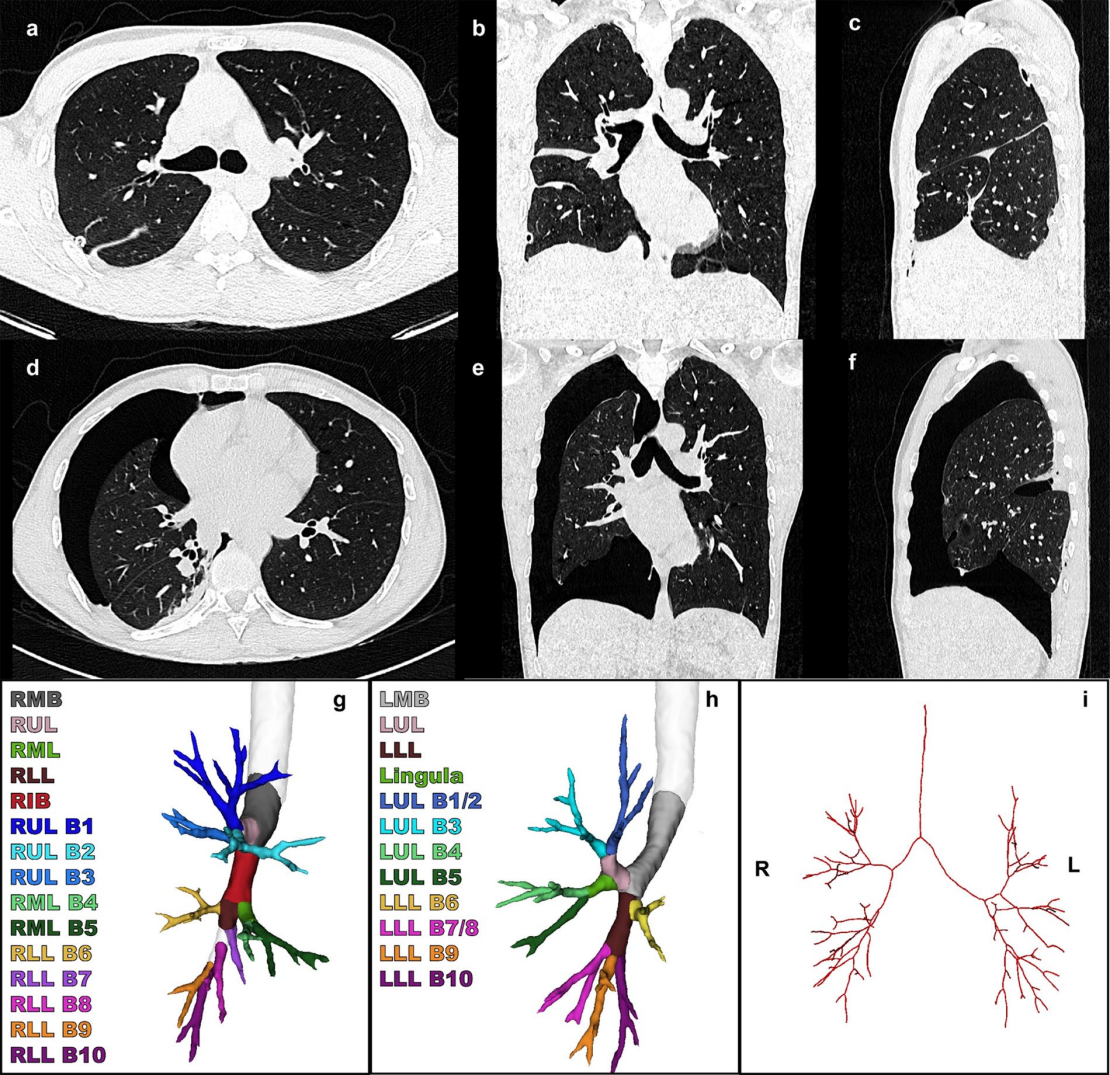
(a) Axial, (b) coronal, and (c) sagittal views of human CT scan in the inspirated state. (d) Axial, (e) coronal, and (f) sagittal views of human collapsed CT scan of the same subject. (g) (h) Bronchus segmentation and (i) bronchus centerline. CT, computed tomography; LLL, left lower lobe; LLL B6, superior segment; LLL B7/8, anterior medial basal segment; LLL B9, lateral basal segment; LLL B10, posterior basal segment; LMB, left main bronchus; LUL, left upper lobe; LUL B1/2, apicoposterior segment; LUL B3, anterior segment; LUL B4, superior lingular segment; LUL B5, inferior lingular segment; RIB, right intermediate bronchus; RLL, right lower lobe; RLL B6, superior segment; RLL B7, medial basal segment; RLL B8, anterior segment; RLL B9, lateral basal segment; RLL B10, posterior basal segment; RMB, right main bronchus; RML, right middle lobe; RML B4, lateral segment; RML B5, medial segment; RUL, right upper lobe; RUL B1, apical segment; RUL B2, posterior segment; RUL B3, anterior segment.
